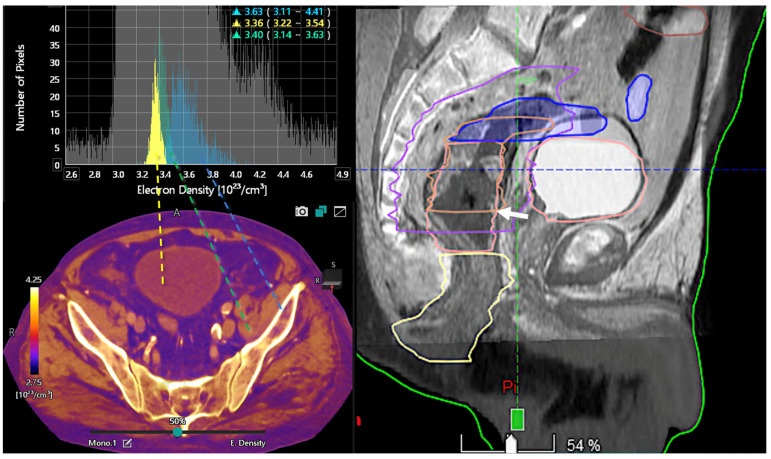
Color-coded electron density map based on DECT (bottom, left). Histogram analysis of electron density values at different locations (bladder lumen [water, yellow], muscle [green], and bone [blue]). Information on electron density is important for radiotherapy treatment planning in order to optimize the dose distribution and volume delineations. Sagittal fused CT and MR image (50% transparency) for therapy planning demonstrates the tumor (white arrow, right image).
